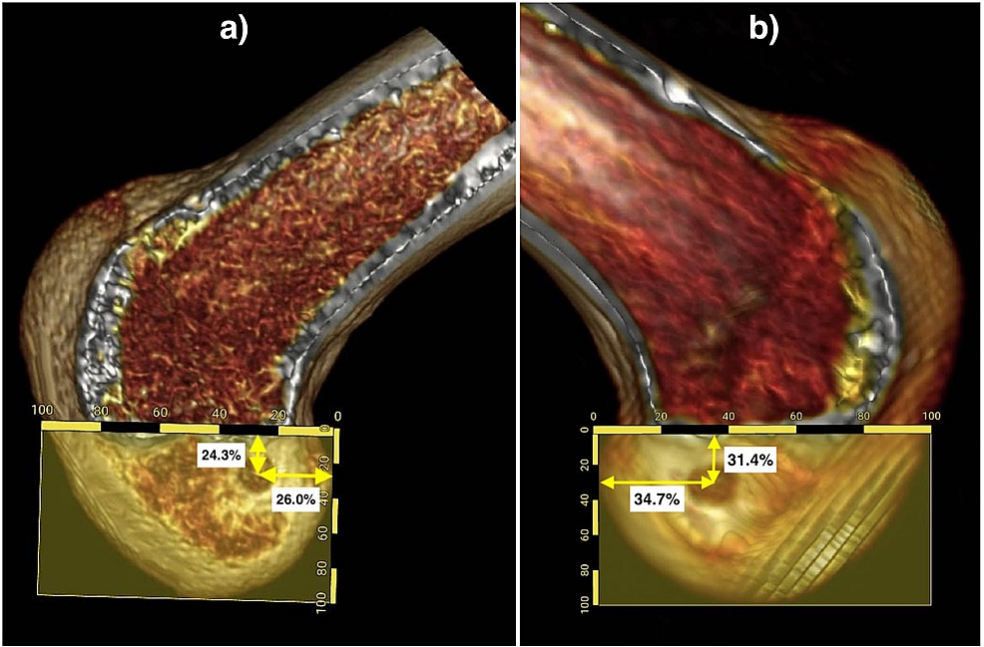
Computed tomography three-dimensional images showing the height and depth of the femoral tunnel entry aperture drilled through the standard anteromedial portal (a), and the far medial portal (b).
